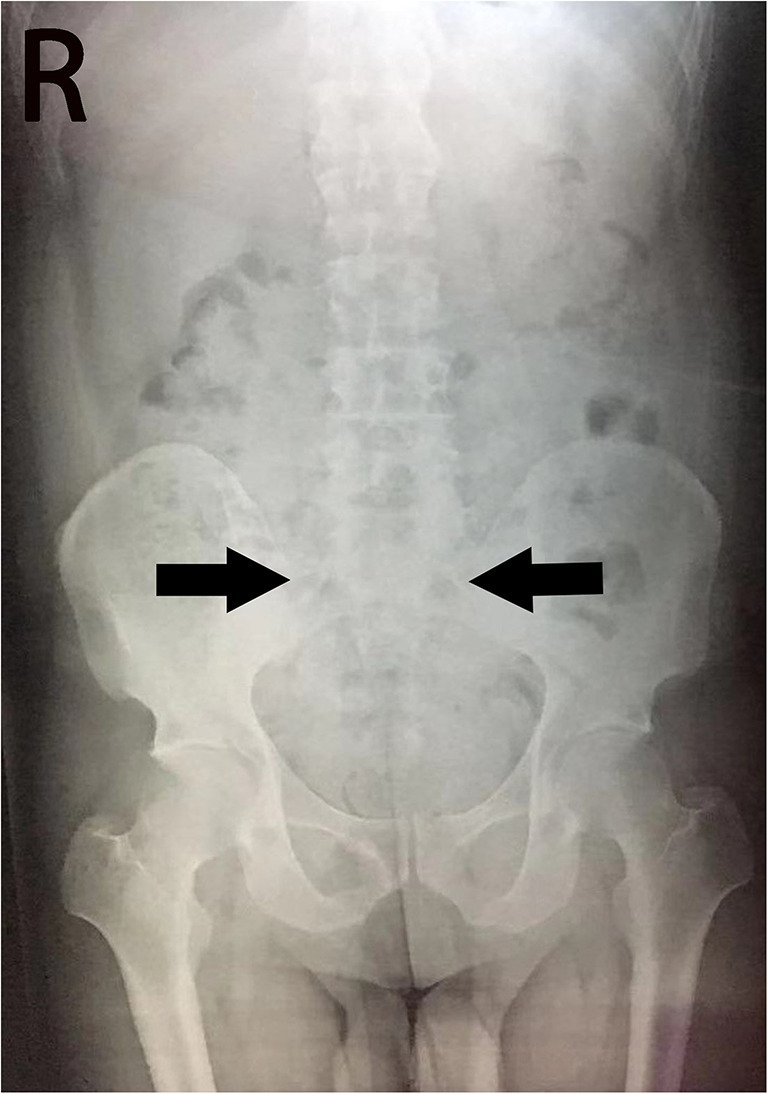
Spinal X-rays of the patient. Spinal x-rays show bilateral sacroiliitis with blurred articular surfaces (arrows), indicating the possibility of ankylosing spondylitis
